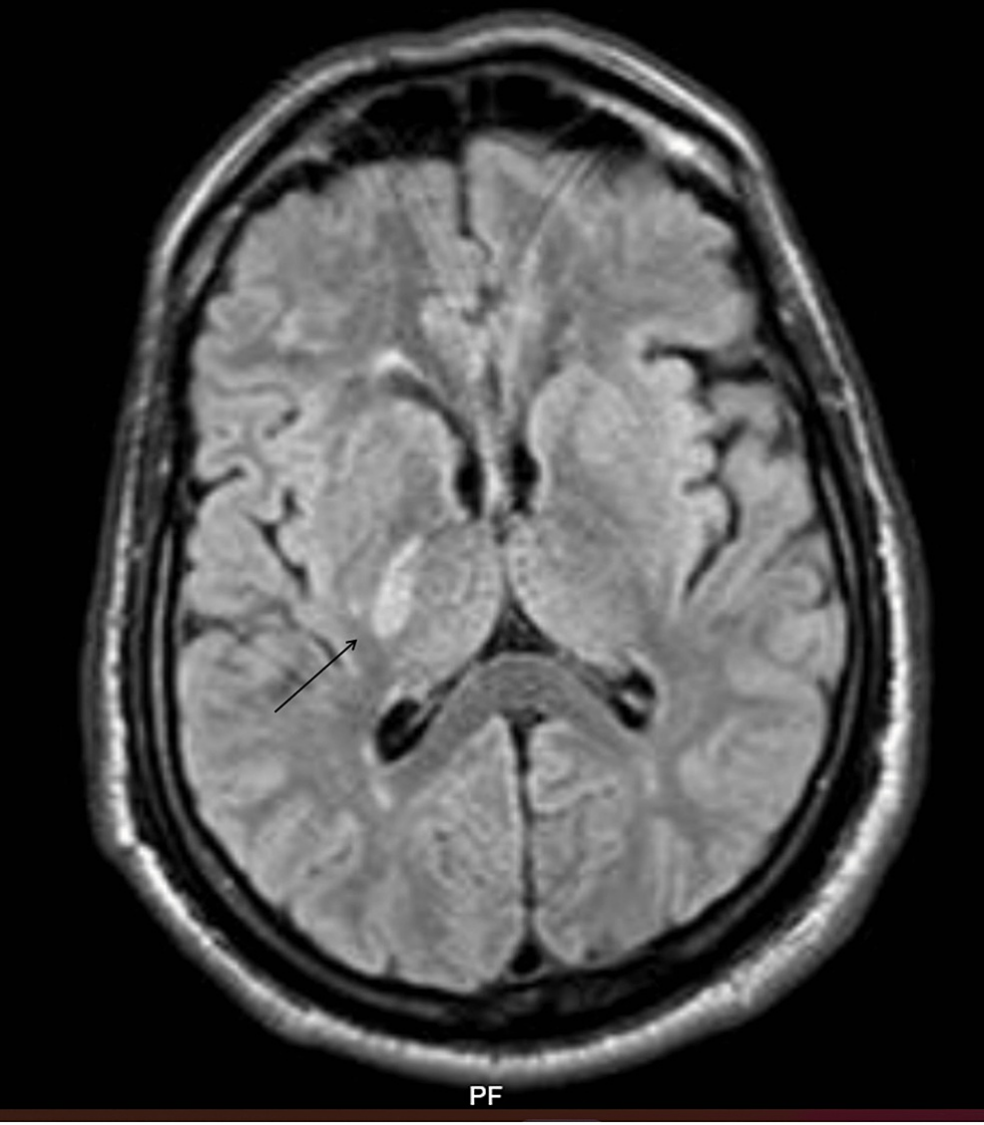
MRI brain during first stroke, three years back showing FLAIR hyperintensity in right capsuloganglionic region (black arrow). MRI: magnetic resonance imaging; FLAIR: fluid-attenuated inversion recovery.
